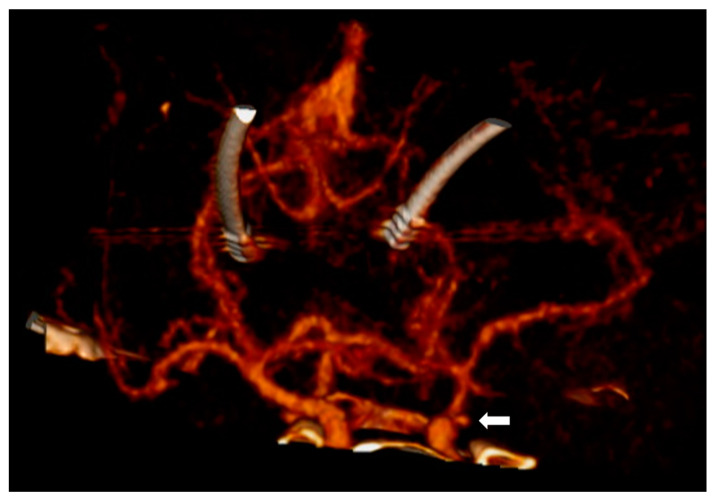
A 3D reconstruction of the CT angiogram showing a supra-ophthalmic aneurysm of the internal carotid artery as the source of hemorrhage (marked by the arrow). DBS leads are displaced to the contralateral side.
